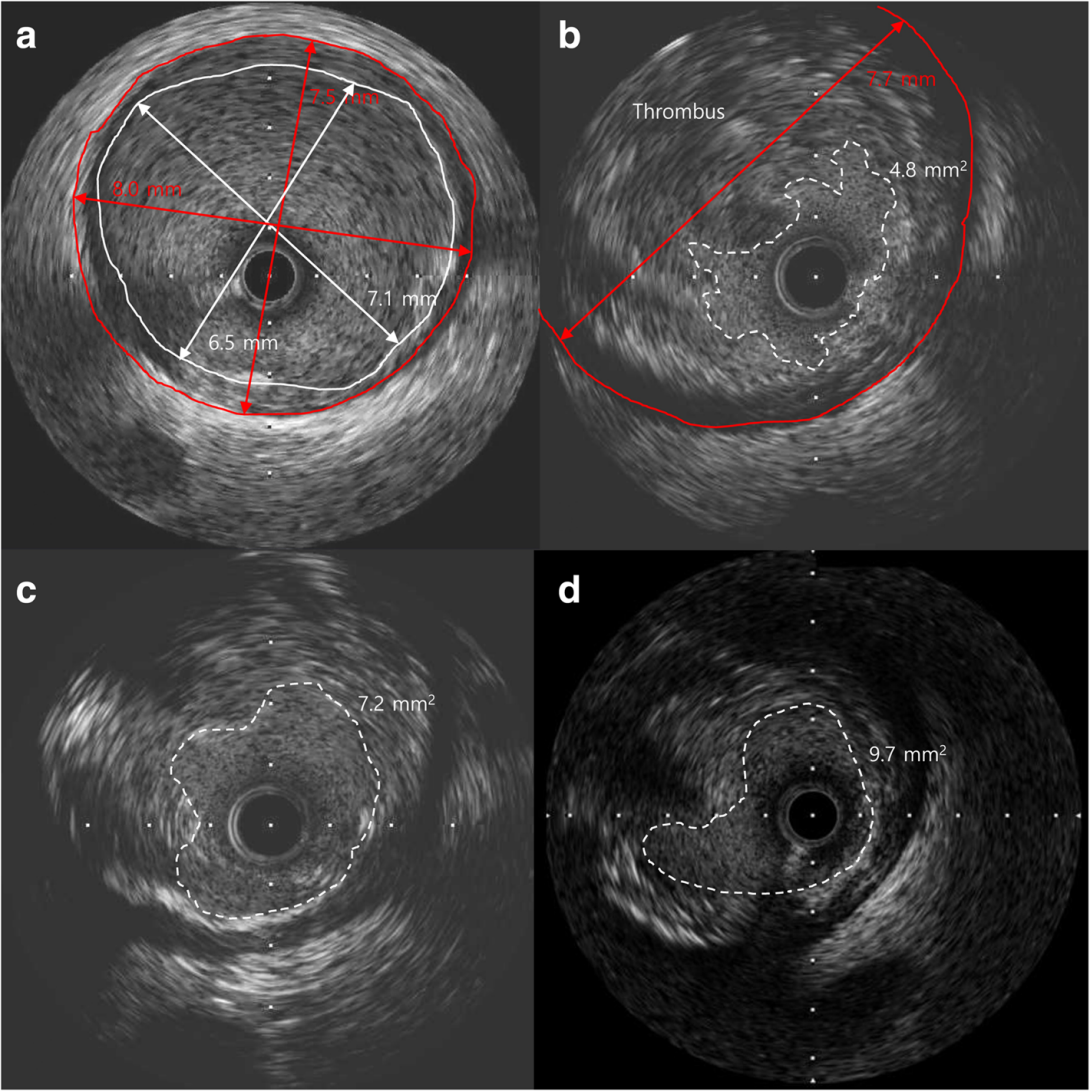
Intravascular ultrasound (IVUS) findings. a Adjacent normal segment proximal to the occlusion site. The shortest EEM and luminal diameter were 7.5 mm and 6.5 mm, respectively. b A ruptured plaque containing many necrotic components and a large amount of thrombus. The diameter of the external elastic membrane was 7.7 mm. c After thrombus aspiration and intracoronary thrombolysis, intermediate stenosis with remnant thrombi was noted. d On the ninth day after the primary percutaneous intervention, a minimal lumen area of about 9.73 mm^2^ with plaque was noted
